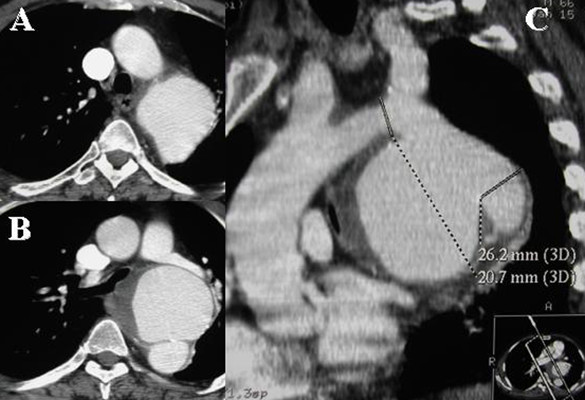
Preoperative CT-A shows an 8-cm saccular aneurysm of the distal arch (A, B); MIP reconstructions shows the involvement of the LSA (C).
